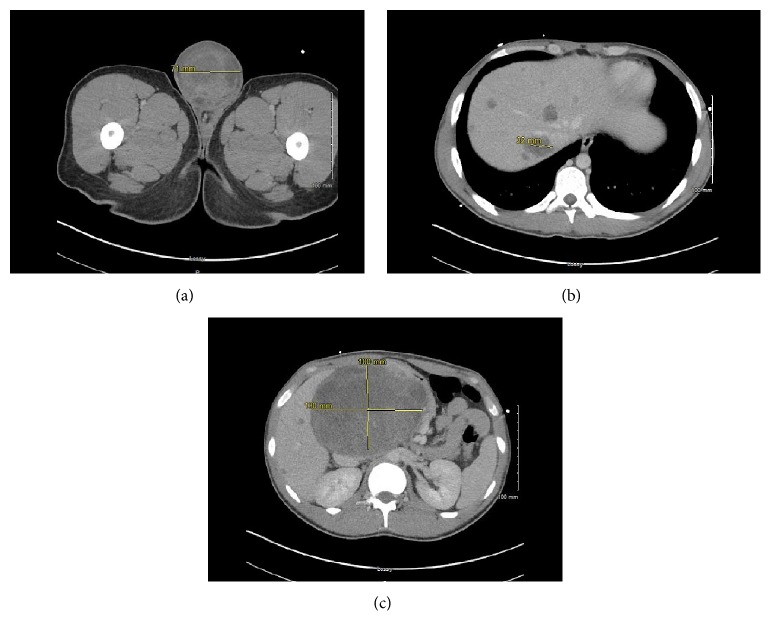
Pelvic computed tomography showing a 9 cm heterogenous mass in right testicle (a). Abdominal CT scan showed multiple hepatic masses (b) and one large heterogenous retroperitoneal mass (c) measuring up to 13 cm in greatest dimension, consistent with metastatic disease.
